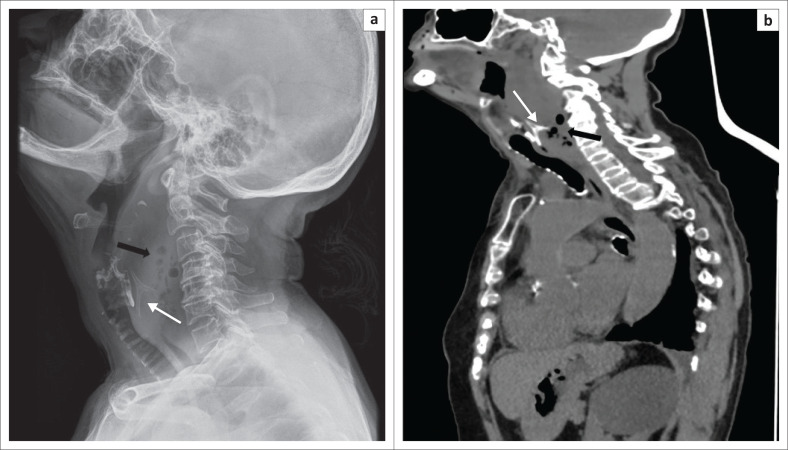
(a) Lateral radiograph of the neck shows a ‘Y’ shaped chicken bone (thin arrow) impacted in the posterior wall of the cricopharynx, along with prevertebral soft tissue thickening demonstrating multiple air locules (thick arrow). (b) Computed tomography sagittal reformatted image confirmed the chicken bone (thin arrow) location and the retropharyngeal abscess.
